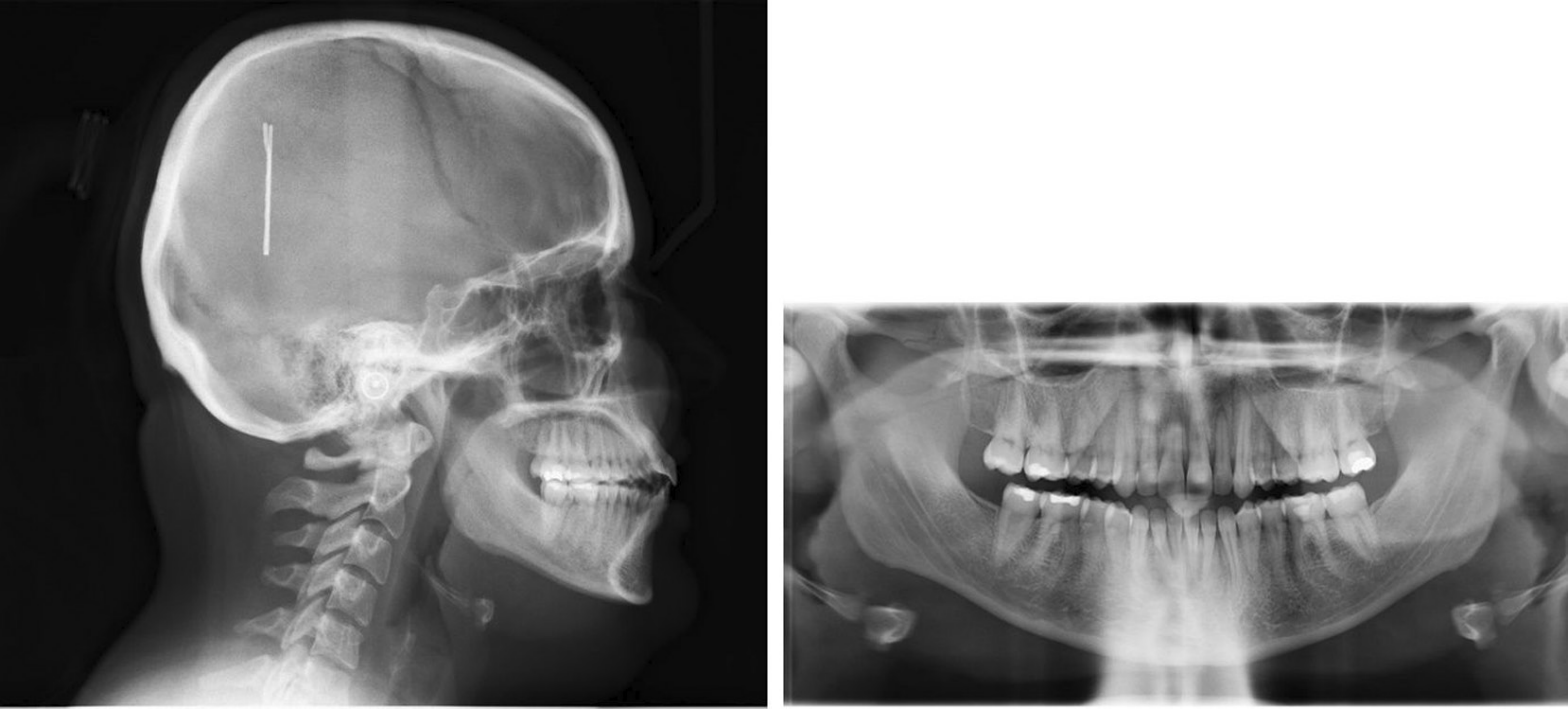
Post‐treatment radiographs
